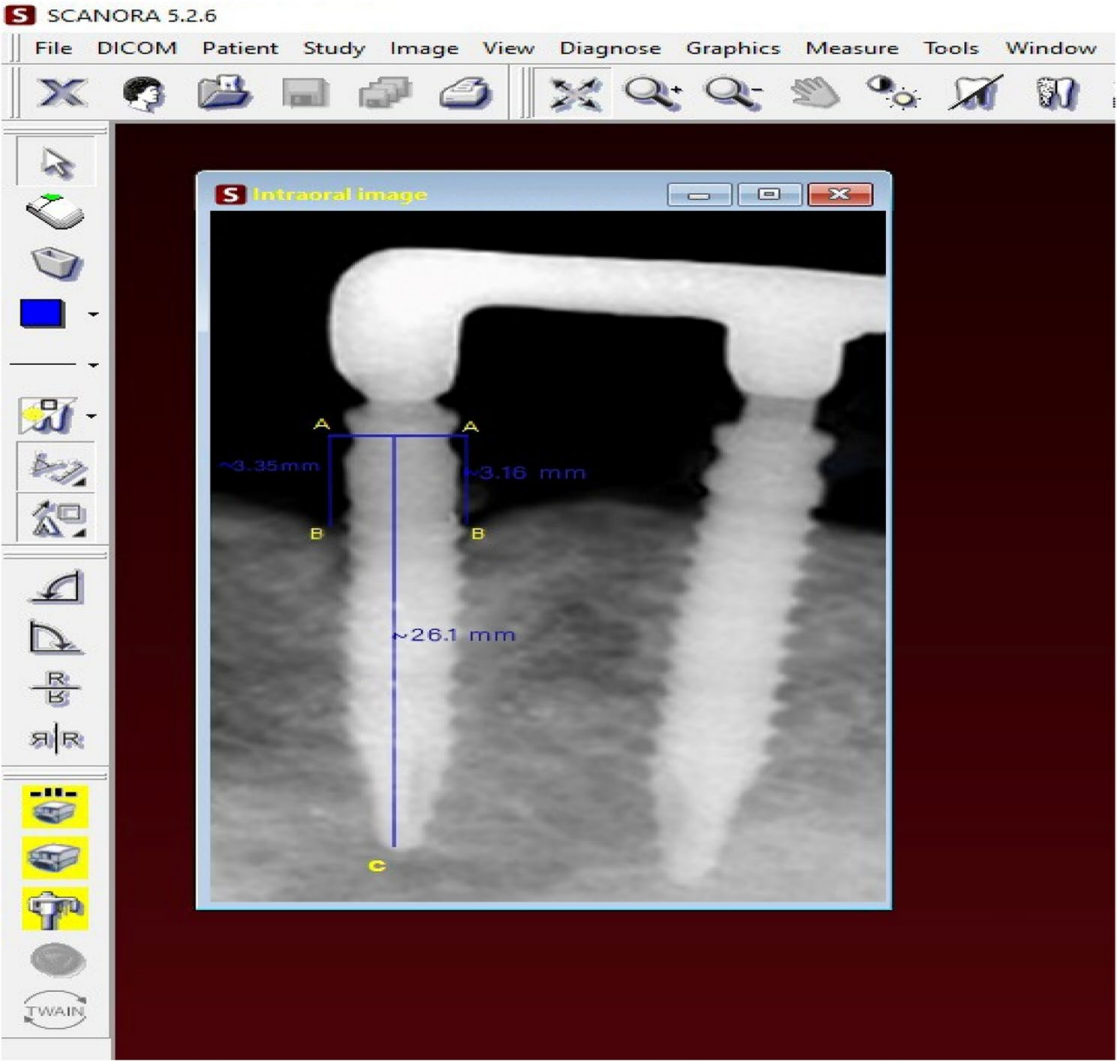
Measurements of the peri-implant vertical bone loss. Measuring implant length in the radiograph. b-Bone height measurement in millimetres as the distance from point A (the implant neck) to point B (the most coronal point of bone-implant contact)
